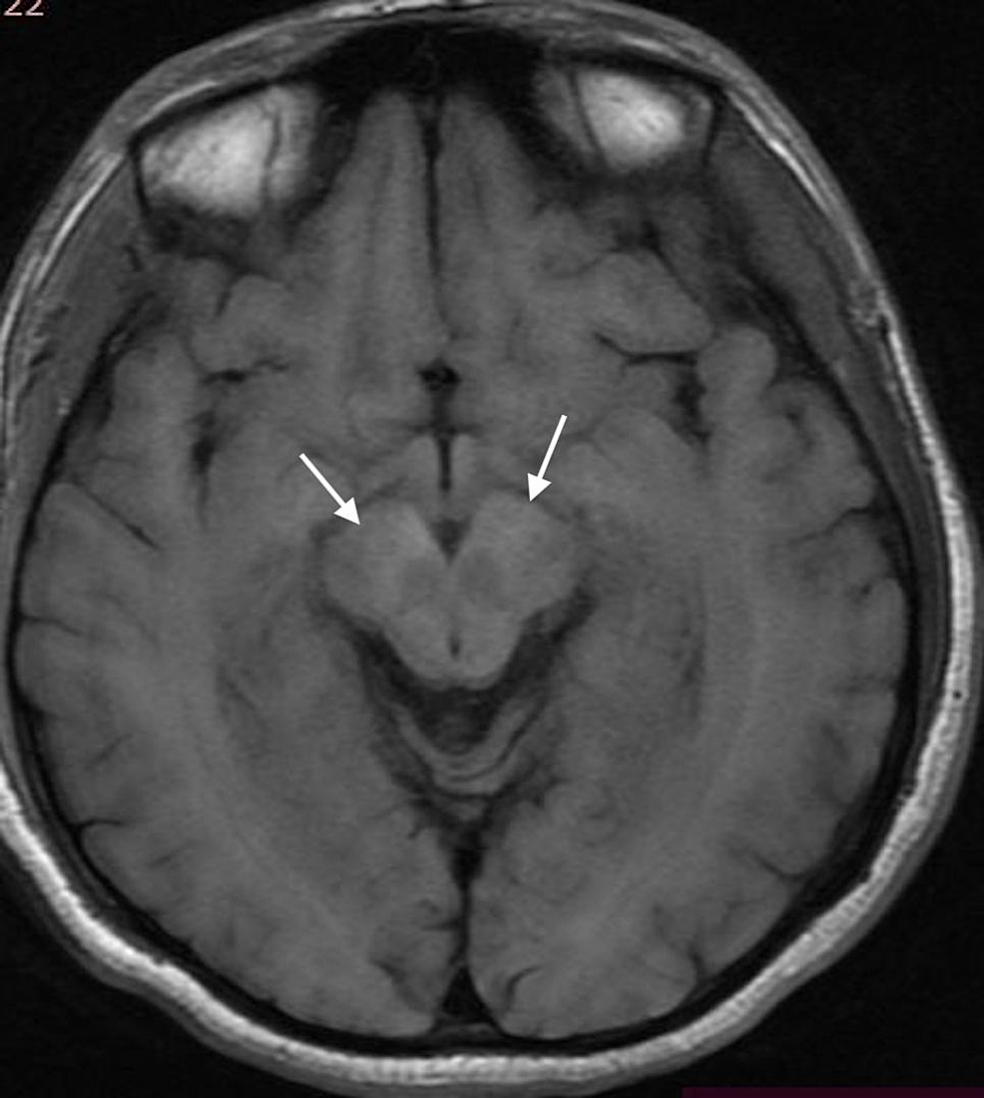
Axial FLAIR MRI image of the brain. MRI FLAIR image of the brain at the level of midbrain showing bilateral hyperintensity on the crura (arrows). FLAIR: Fluid-attenuated inversion recovery.
